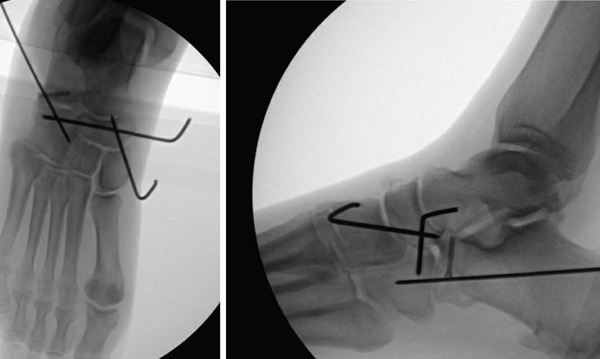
Intraoperative anteroposterior and lateral radiograph of anterior–inferior calcaneal process using Kirschner wire stabilization followed by 2.5‐mm compression screw.
